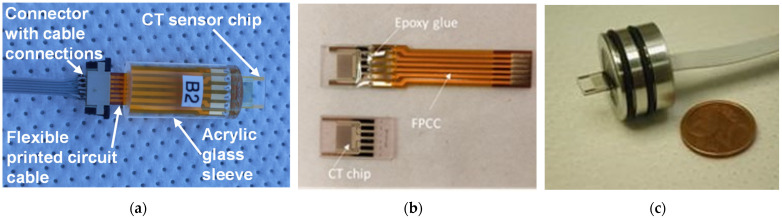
CT sensor encapsulated in different housings: (a) and (b) for laboratory measurements and characterization and (c) for deep-ocean deployment (2000 m).
